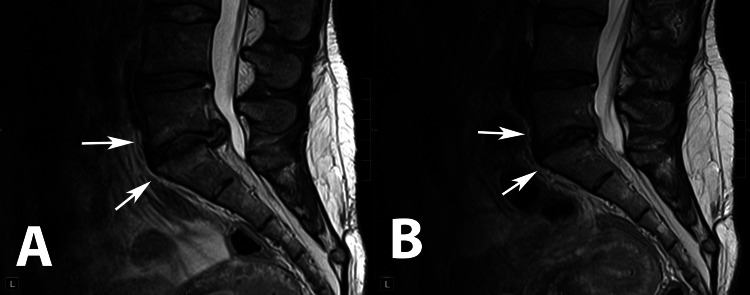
Sagittal T2-weighted MRI images of Patient 1 showing the spine at initial presentation (A) and one year after endoscopic discectomy (B). Evident partial resolution of Modic changes in adjacent L5-S1 vertebral endplates (white arrows).
